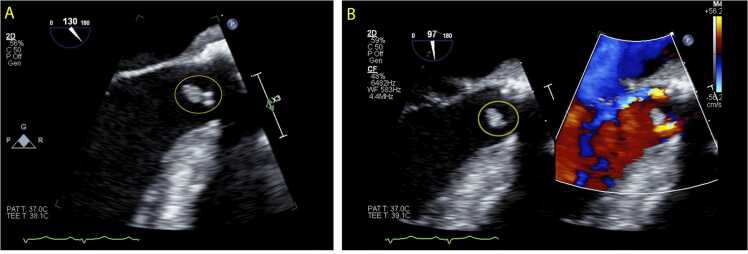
Transesophageal echocardiogram long axis views (A,B) show a 1.3 cm mobile vegetation on the aortic valve, prior to the initiation of antibiotics.
